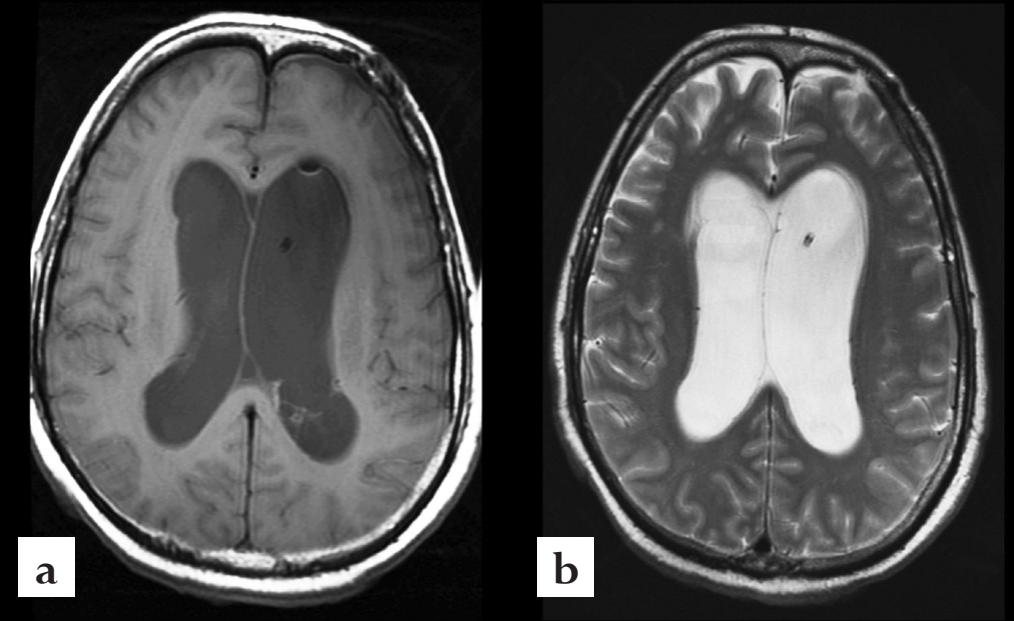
Case #1. (a): Axial T1 weighted magnetic resonance imaging of the brain without contrast showing no intraventricular lesions. (b): Axial T2 weighted magnetic resonance imaging showing ventriculostomy catheter in the body of left lateral ventricle.
